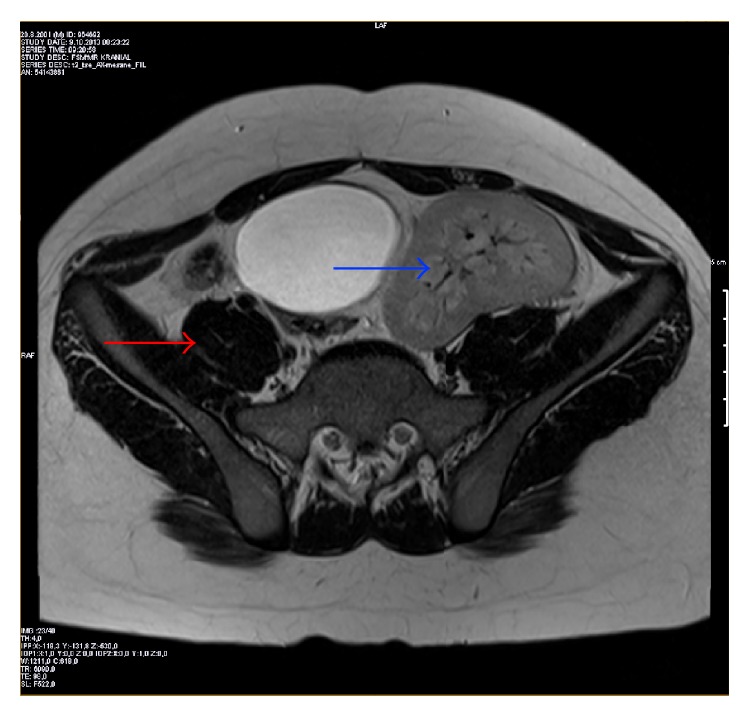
Magnetic resonance imaging of abdomen on the axial plane. There were right renal agenesis (red arrow) and left ectopic pelvic kidney in the neighborhood of urinary bladder (blue arrow).
